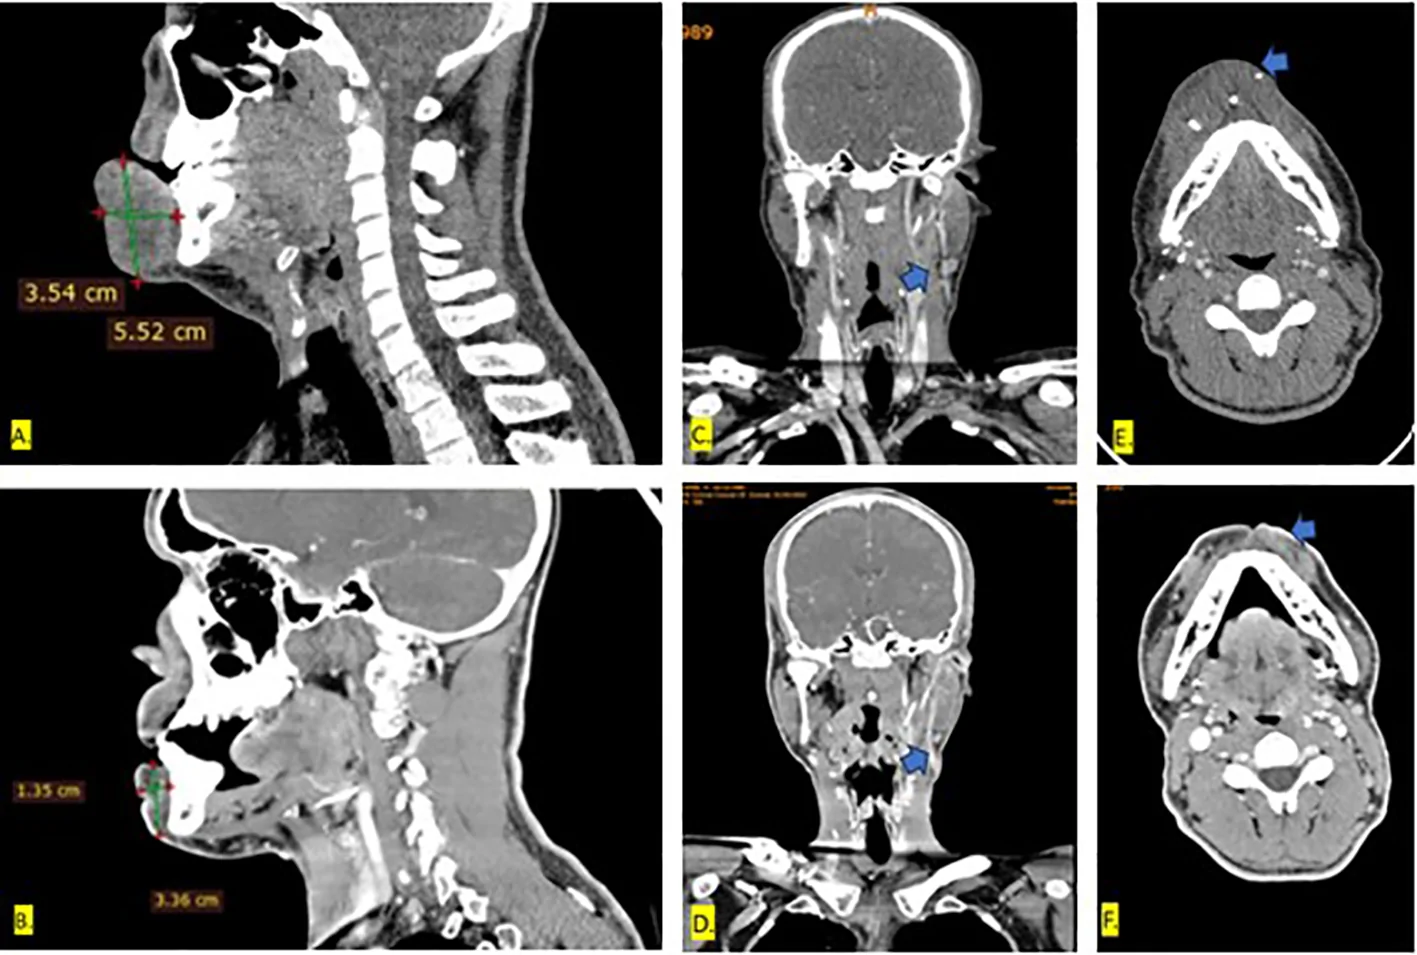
CT imaging demonstrating response to treatment in desmoplastic melanoma. (A) Baseline imaging prior to IDCT initiation demonstrating a large heterogeneous mass involving the lower lip and anterior mandibular region measuring 5.52×3.54 cm. (B) Six months following treatment initiation showing complete resolution of the previously identified mass with restoration of normal lip contour. The lower lip appears flat with normal soft tissue density and no evidence of residual tumor. (C) Pre-IDCT Coronal CT of the head and neck shows a cervical lymph node with ovoid morphology in the left parotid space and ipsilateral level IV cervical region (pointed). (D) Post-treatment image shows decreased lymph node size (pointed). (E) Pre-treatment Axial CT of the lower lip and chin demonstrates an ovoid soft tissue mass with heterogeneous density, hypodense center, peripheral soft tissue density, and mild peripheral enhancement (pointed). (F) Post-treatment image shows interval reduction in mass size (pointed).
